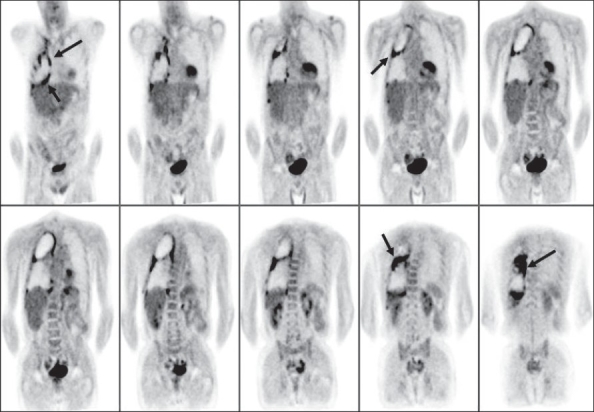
Thirteen millimeter coronal PET images in a 64-year-old man with an abnormal CT scan of the chest. The patient had a history of talc pleurodesis for recurrent right pleural effusion, which is responsible for the intense pleura-based FDG activity around the right lung
